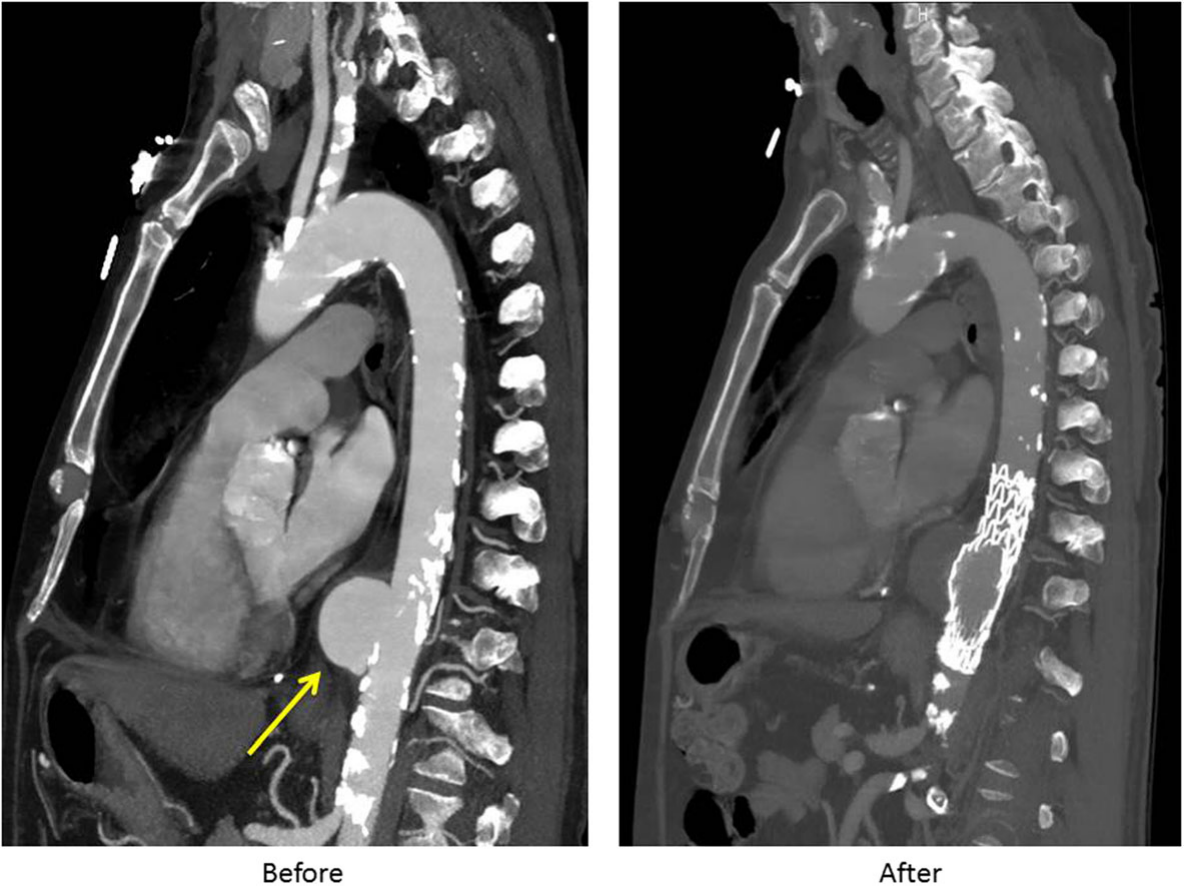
Computed tomography angiography in sagittal section of descending thoracic pseudoaneurysm caused by blunt aortic injury before (left) and after (repair) with TEVAR
